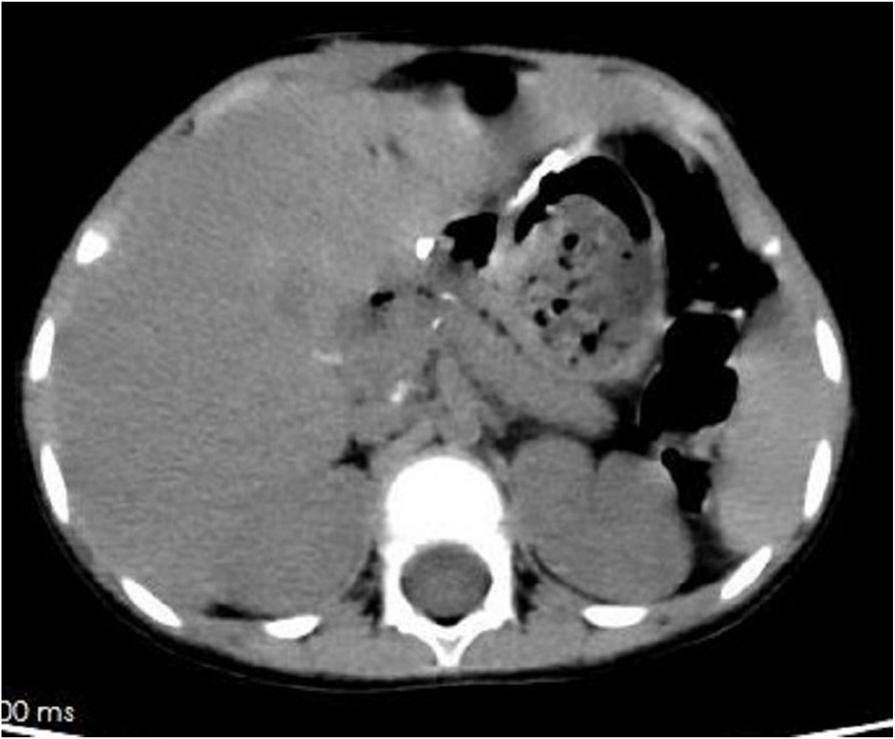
Abdominal CT showed no recurrence 3 months after the operation
